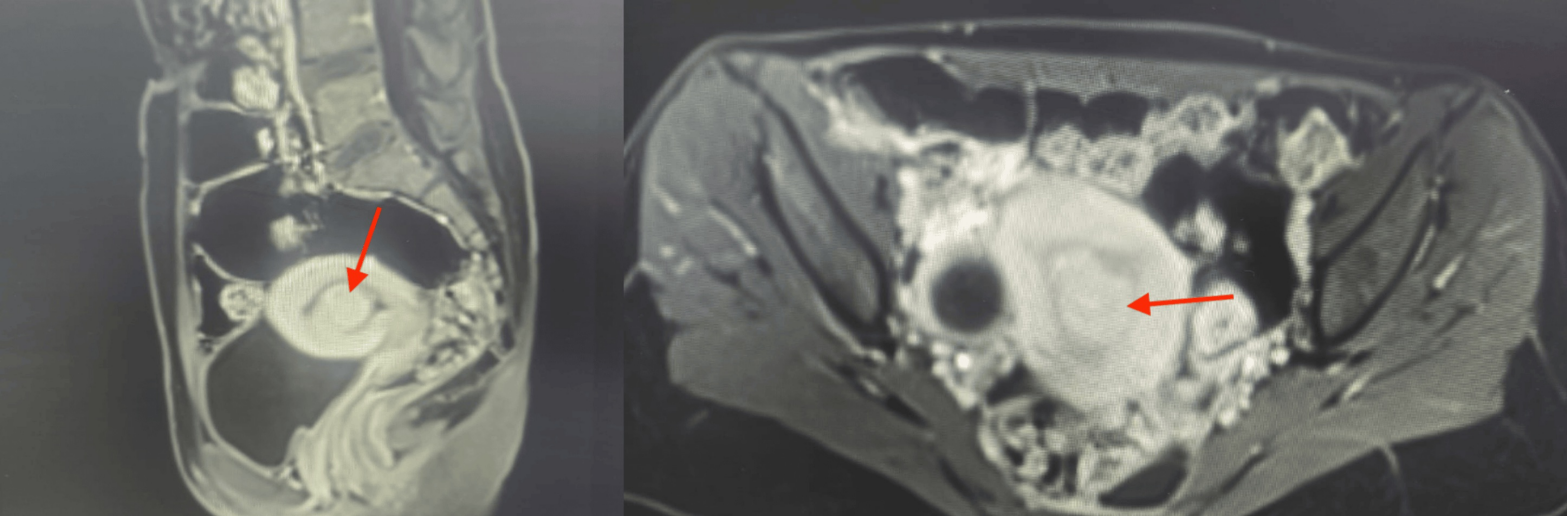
MRI images of the fibroids; the red arrow depicts the submucosal fibroid.
